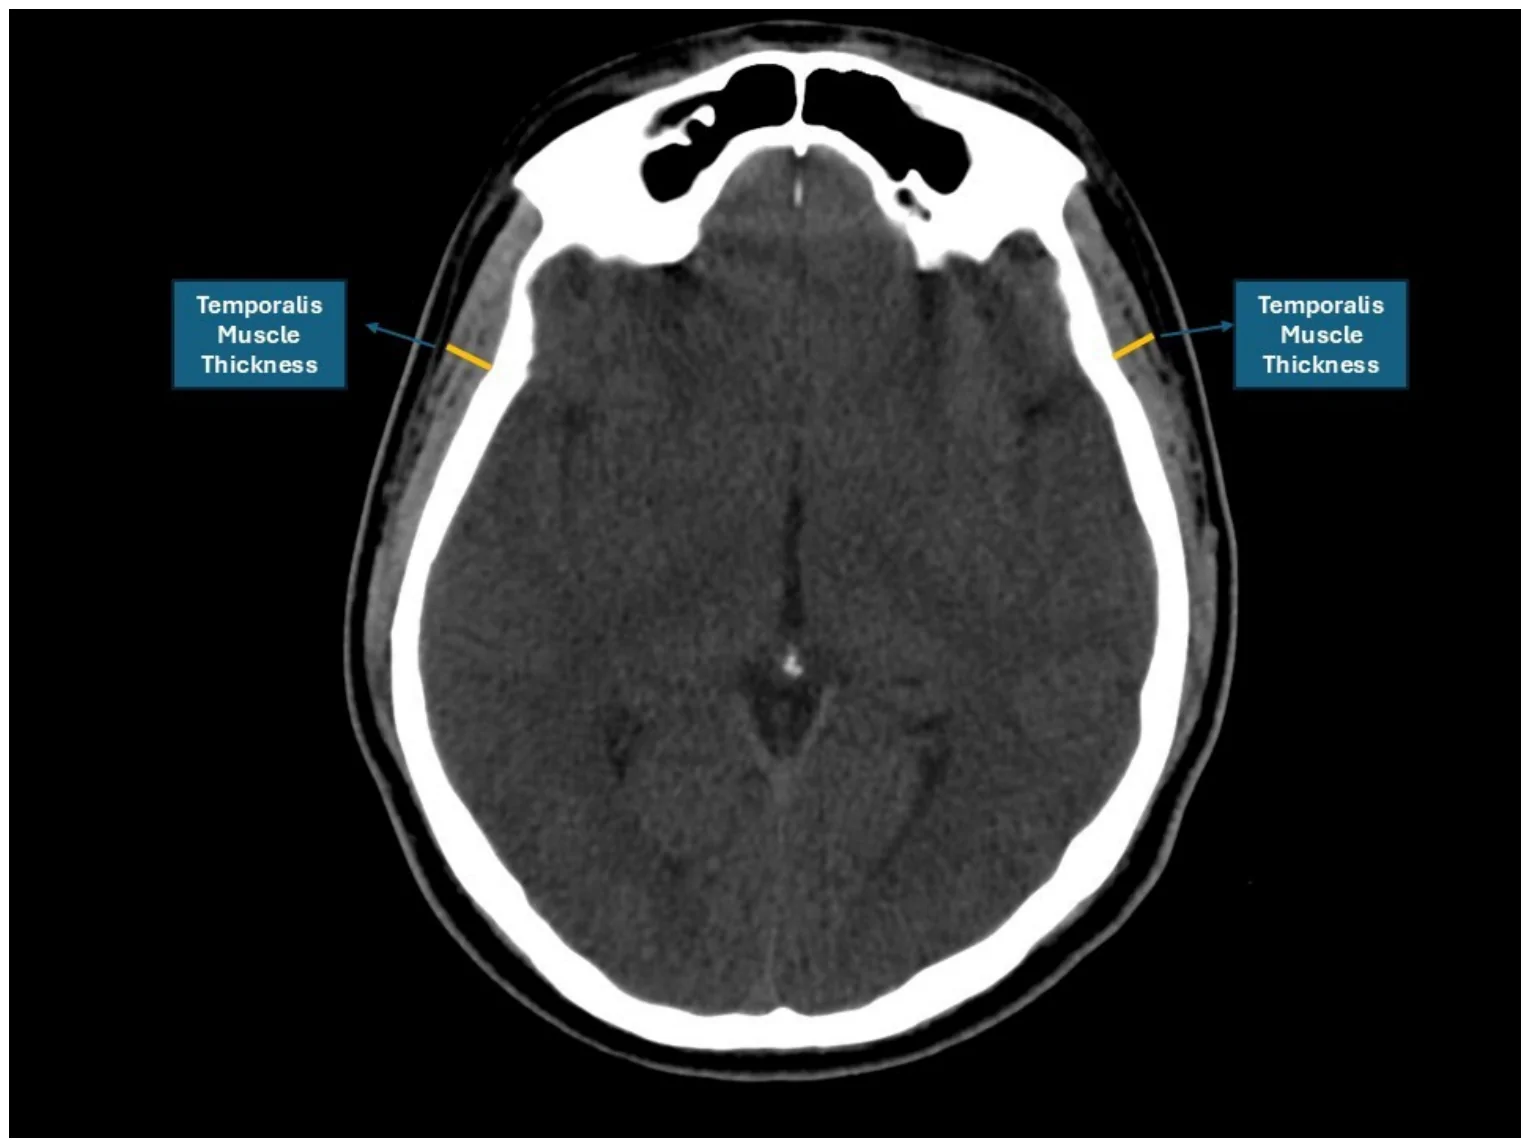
Representative axial non-contrast brain CT image demonstrating bilateral temporalis muscle thickness measurement. Measurements were obtained manually at a level approximately 0.5 cm above the superior orbital rim adjacent to the Sylvian fissure, using a window setting of 20–200 Hounsfield units.
